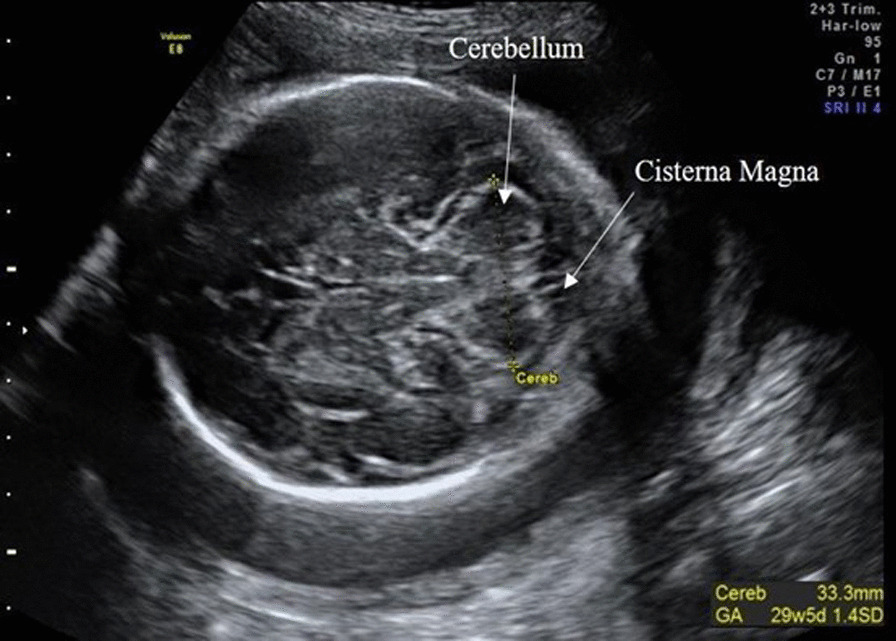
Example of the TCD measurement in a 27-week plus 6-day old female fetus. For TCD measurements, the transducer was slightly rotated from the thalamic plane (used to measure HC) until the posterior fossa was visible. TCD was measured using the ‘outer-to-outer’ method. TCD taken during the research scan always showed LGA. This is due to the fact that the internal growth charts on the GE Voluson 8 Expert ultrasound scanner [60] did not cover this GA. However, all TCD were within expected 5–95 centile ranges
